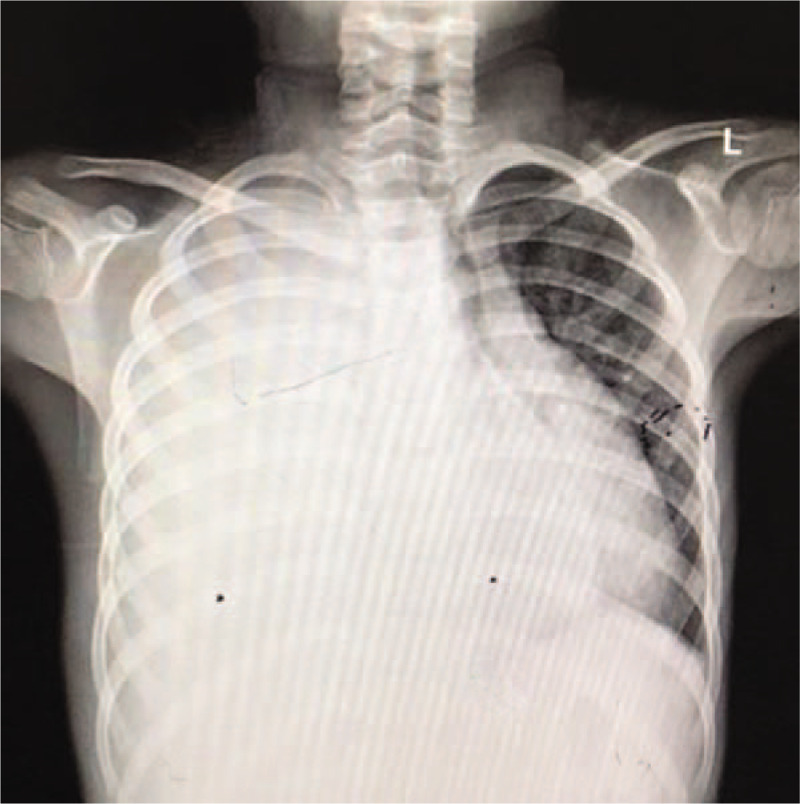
Chest X-ray showed massive pleural effusion in the right thorax.
